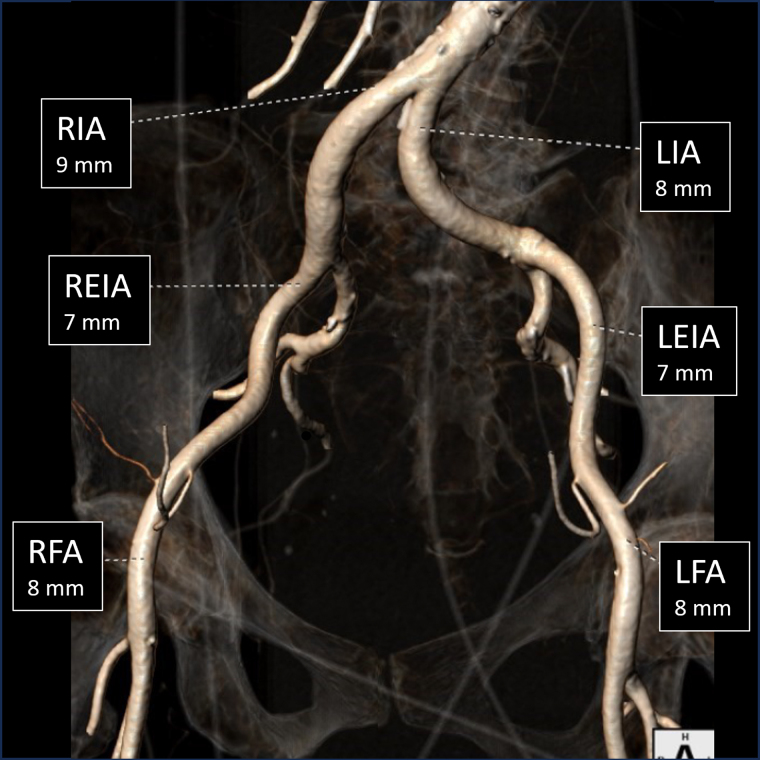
Computed tomography angiography of peripheral accesses. 3D reconstruction of the iliac and femoral arteries displaying access practicability. RIA, right iliac artery; REIA, right external iliac artery; RFA, right femoral artery; LIA, left iliac artery; LEIA, left external iliac artery; LFA, left femoral artery.
